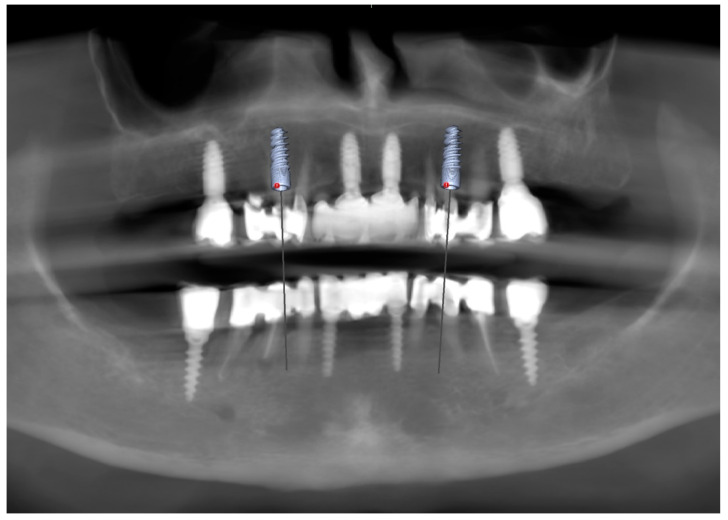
Preoperative panoramic reconstruction from CBCT, showing the initial condition of the existing tooth- and implant-supported restorations in the maxilla and mandible, as well as the visualisation of two planned implants in positions 14 and 24.
